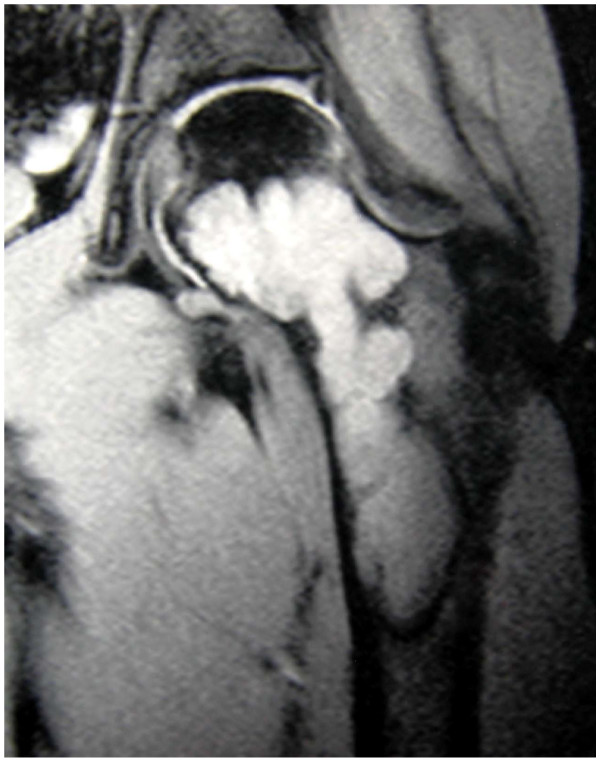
MRI, preoperative: Cystic lesion of the proximal femur.
